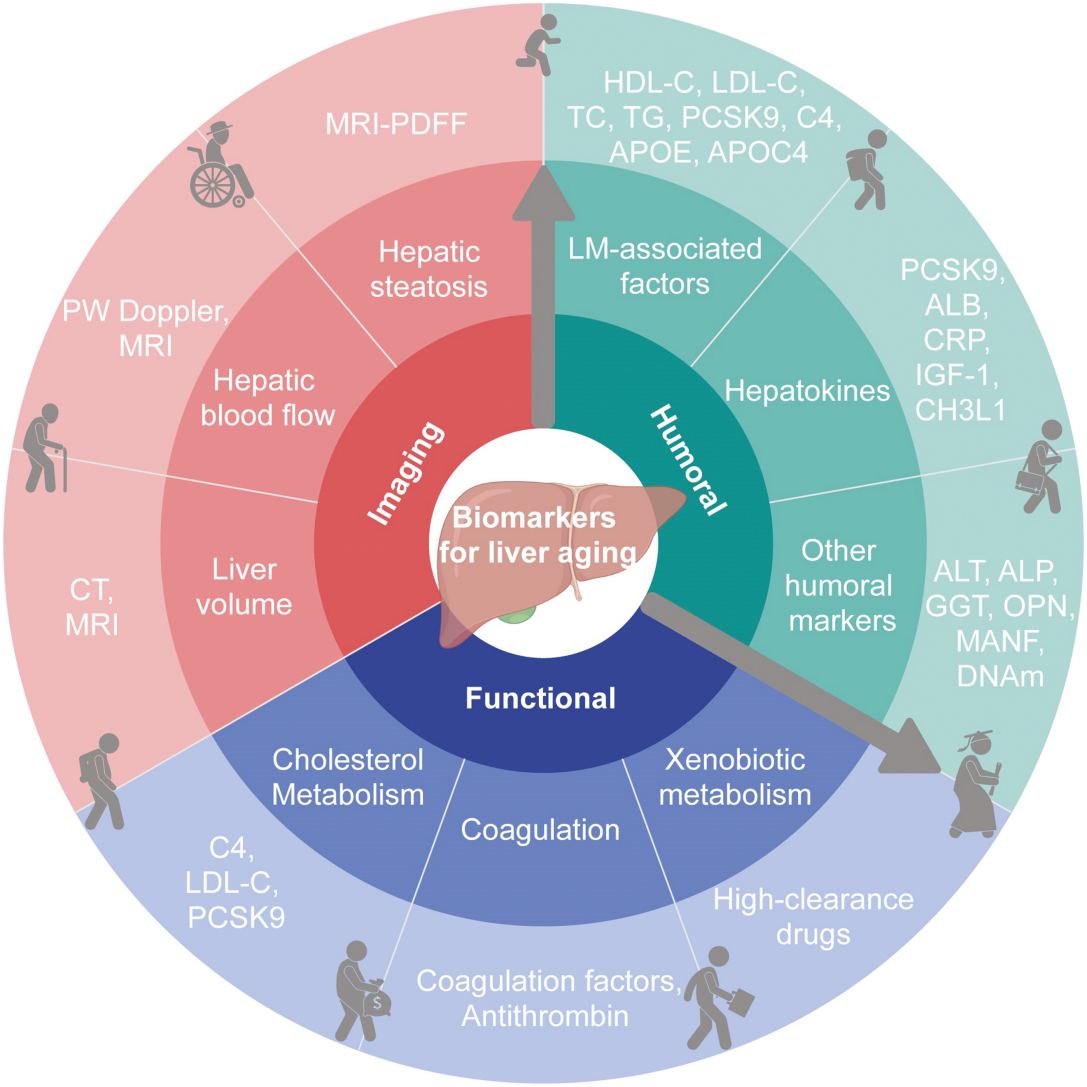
Framework of biomarkers for liver aging. The proposed framework for liver aging consists of three dimensions: functional, imaging, and humoral biomarkers. Abbreviations: ALP, alkaline phosphatase; ALT, aminotransferase; APOC4, apolipoprotein C4; APOE, apolipoprotein E; C4, 7α-hydroxy-4-cholesten-3-one; CHI3L1, chitotriosidase-3-like protein 1; CRP, C-reaction protein; CT, computed tomography; DNAm: DNA methylation; GGT, gamma-glutamyl transferase; HC II, heparin cofactor II; HDL-C, high-density lipoprotein cholesterol; IGF-1, insulin-like growth factor 1; LDL-C, low-density lipoprotein cholesterol; LM, lipid metabolism; MANF, mesencephalic astrocyte-derived neurotrophic factor; MRI, magnetic resonance imaging; OPN, osteopontin; PCSK9, proprotein convertase subtilisin/kexin type 9; PDFF, proton density fat fraction; PW Doppler, pulsed-wave Doppler; TC, total cholesterol; TG, triglycerides.
